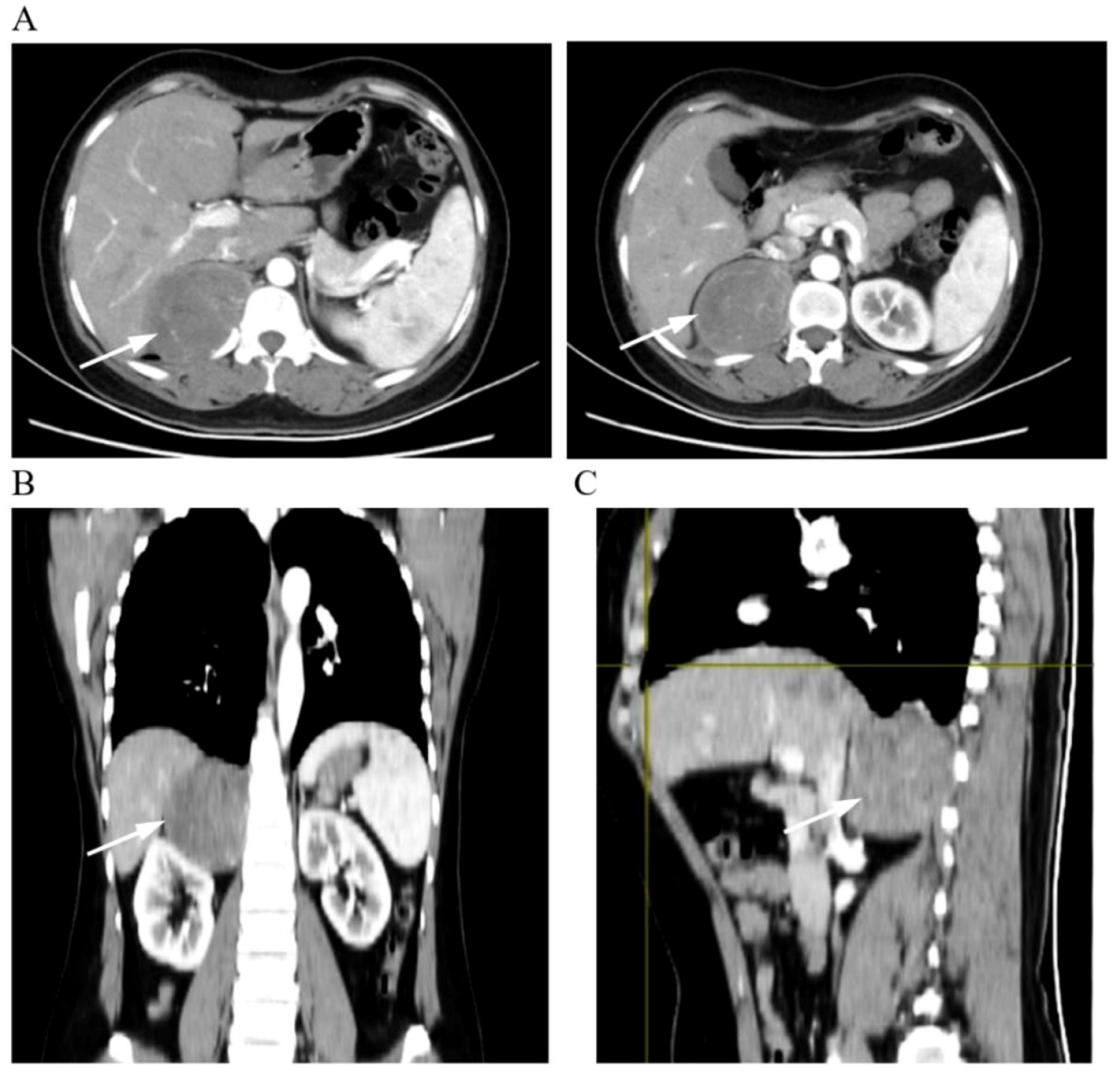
Representative images of CT scans. (A) Cross-sectional enhanced scan. (B) Coronal scan. (C) Sagittal scan. The arrows in the picture show the tumor tissue.
